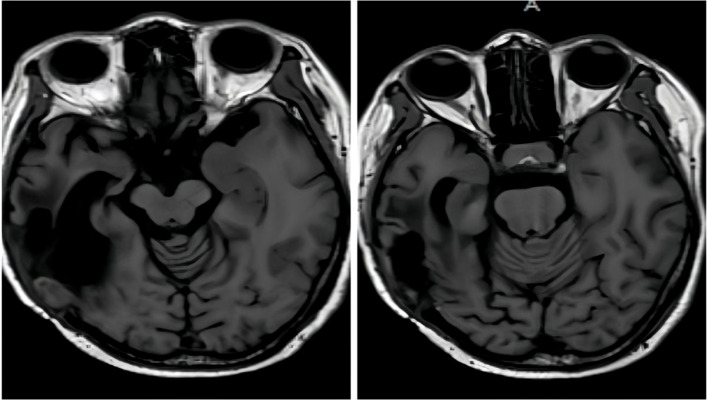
Post-treatment MRI (T1-weighted) after combined Gamma Knife and bevacizumab therapy revealed no significant abnormal signal intensity.
